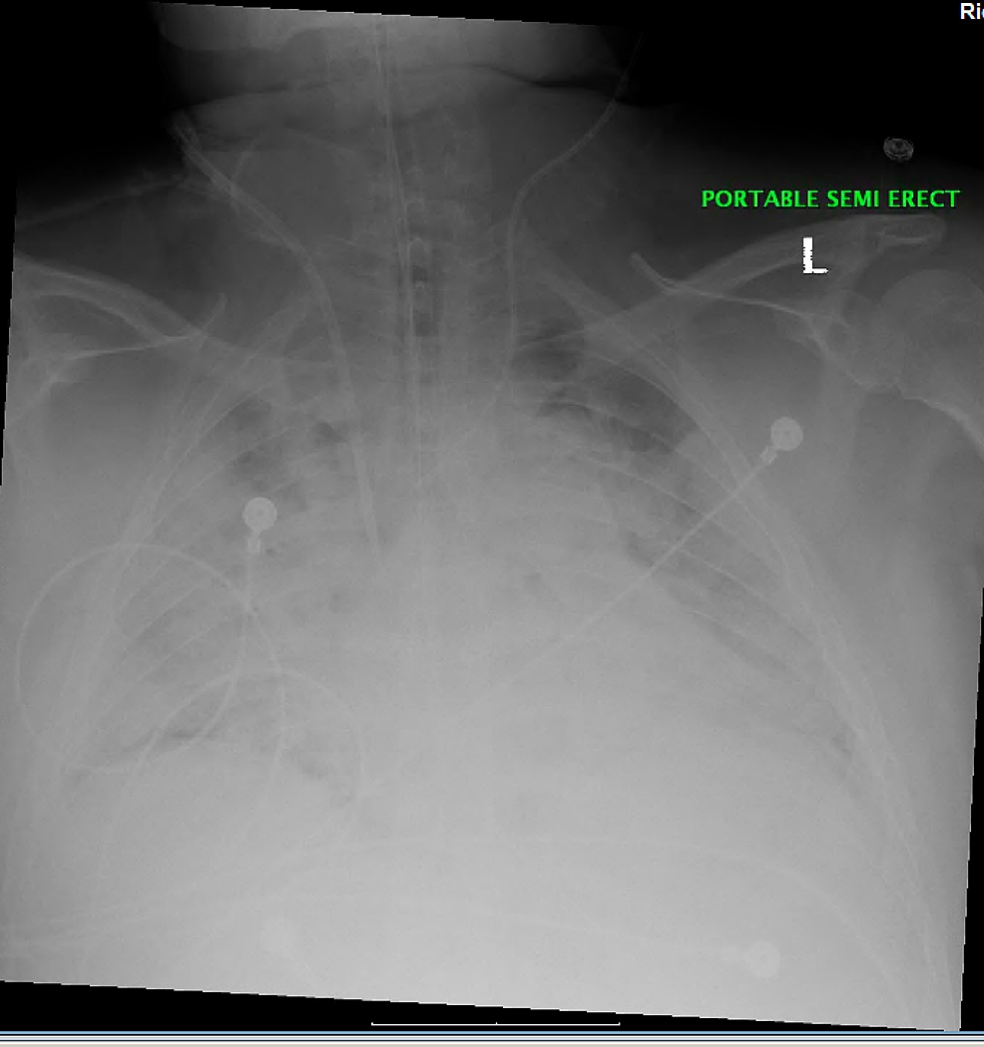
CXR exhibiting ARDS. CXR: chest X-ray; ARDS: acute respiratory distress syndrome.
